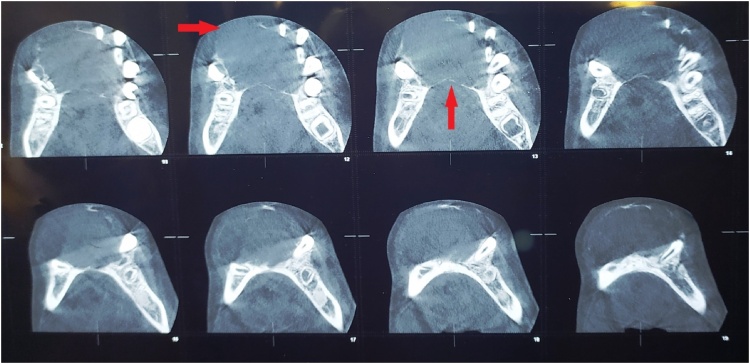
CT scan (axial cut) picture of the patient showing iso-dense cystic mass (arrow).
